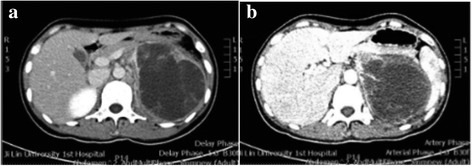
Computed tomography (CT) scans showing a mixed solid and cystic lesion (a) that was enhanced on contrast-enhanced CT (b)
